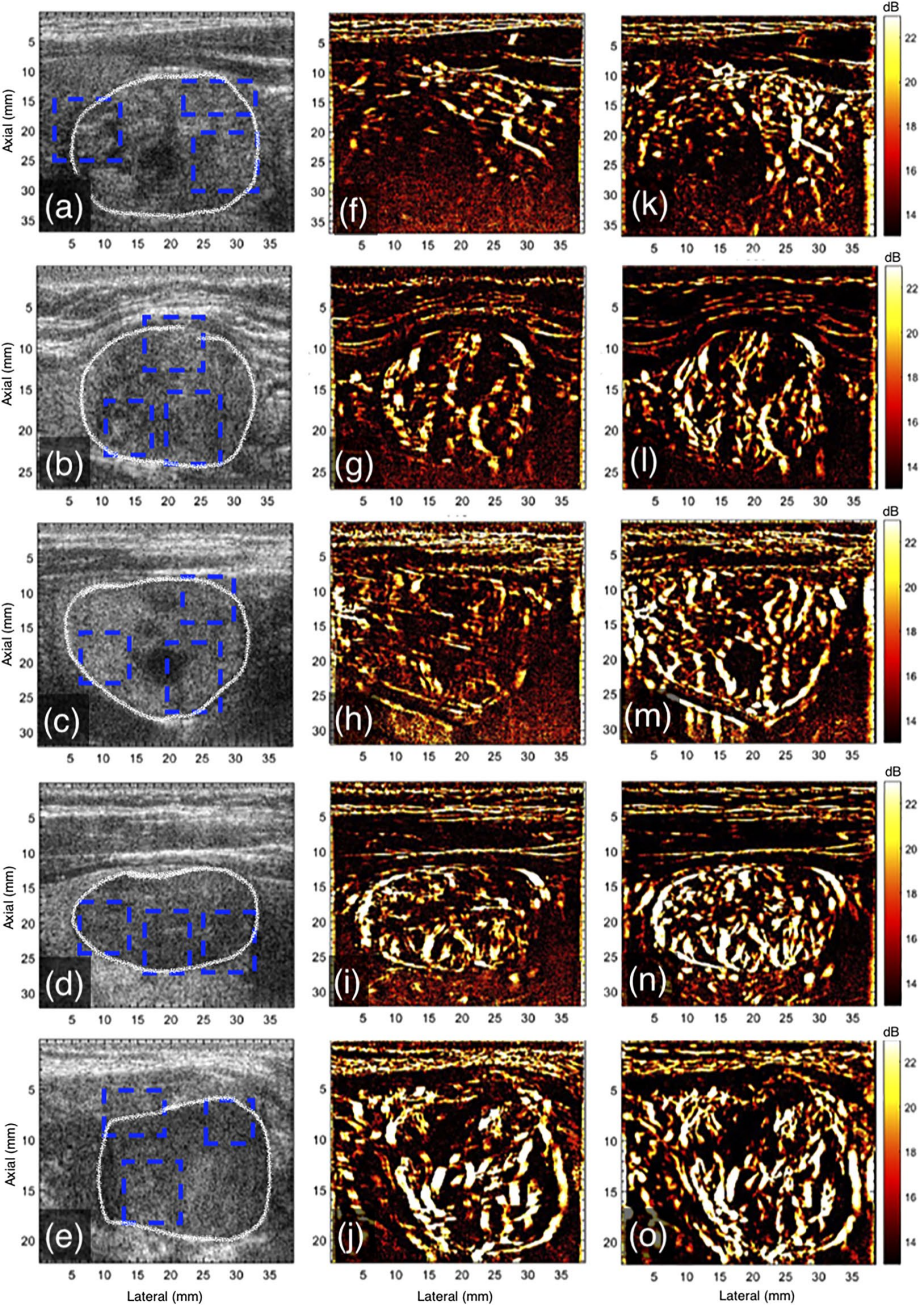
Displays the plane wave B-mode sonograms (a–e) and the corresponding PD images without (f–j) and with (k–o) motion correction, for patients 3–7, respectively. The outline of the thyroid nodules is indicated in white in the B-mode sonogram (a–e). For the sake of clarity in visualizing the small blood vessel structures in the PD images, the blue ROIs that were used for quantitative analysis in Fig. 9 were indicated in the B-mode sonograms.
